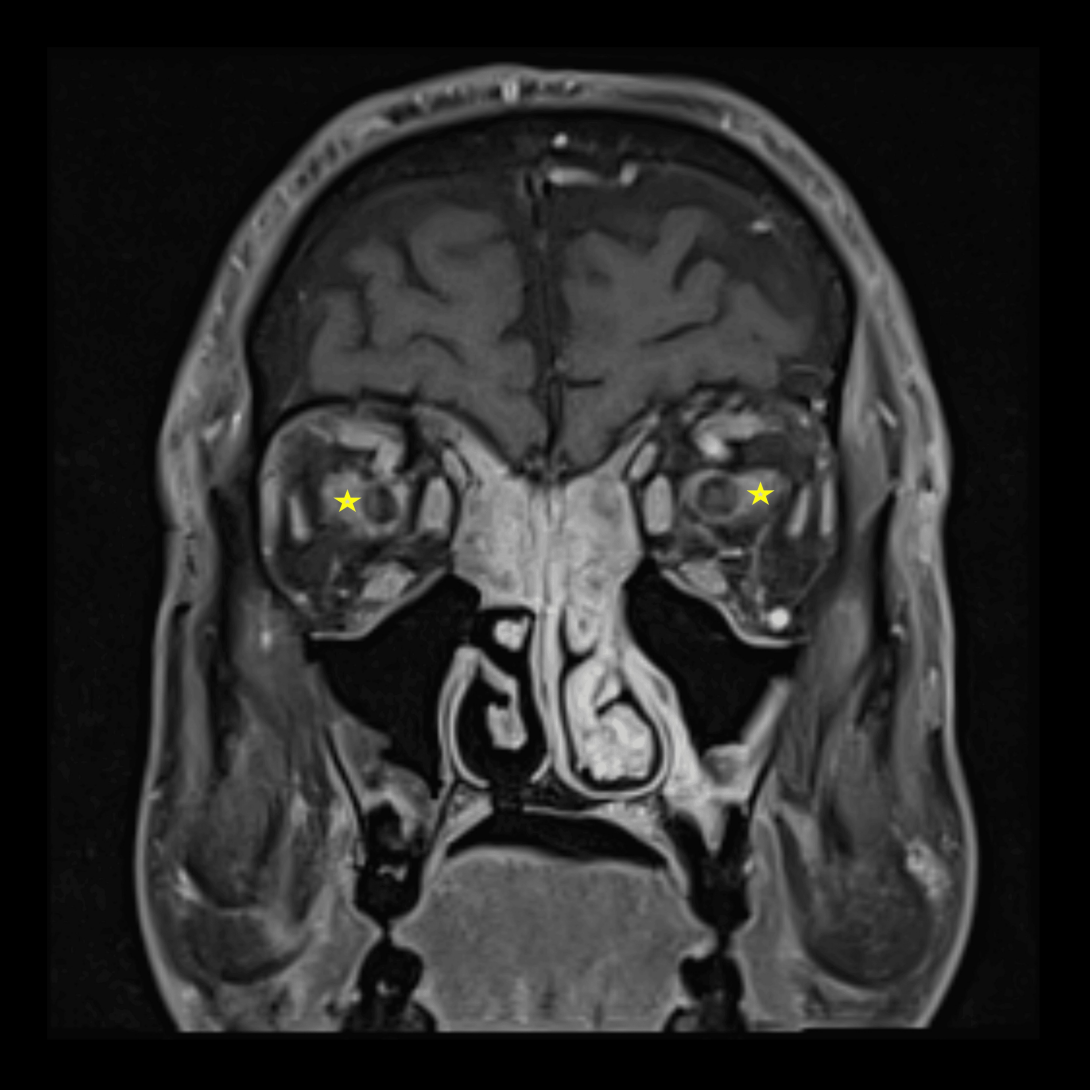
Magnetic resonance imaging of the orbits with stranding of the soft tissue fat near optic nerve insertions (stars).
